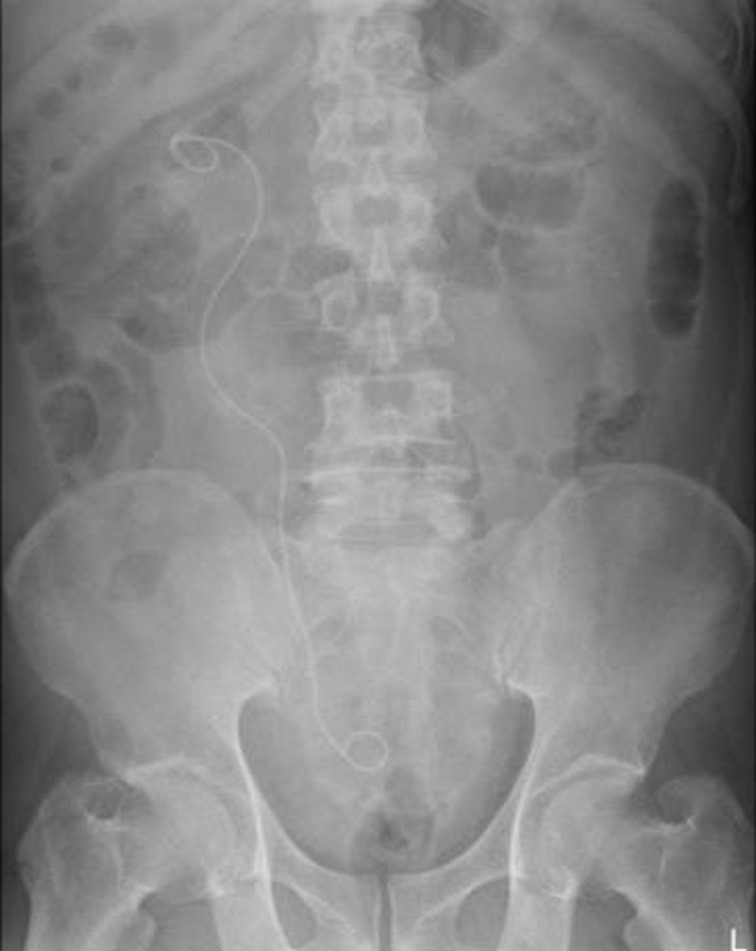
representative image of abdiminal radiograph after successful DJ stent insertion
